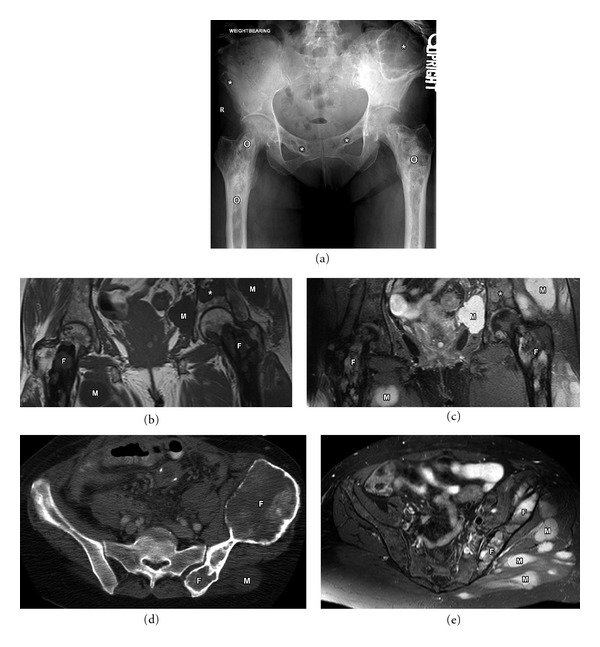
61-year-old female with Mazabraud syndrome (polyostotic fibrous dysplasia and soft tissue myxomas (M)). Plain film (a) demonstrates multiple lucent lesions with ground glass matrix in the pelvis (asterisk) and long predominantly sclerotic abnormality in bilateral femurs (circle). Fibrous dysplasia is often described as a long lesion in the long bone. Coronal T1 (b) and STIR (c) MRI images demonstrating multiple fibrous dysplasia (F) lesions. Note that some of these maintain low to intermediate signal intensity on both T1 and T2 images (asterisk). Axial CT (d) and axial T2 fat sat (e) MRI images demonstrating contour abnormality of the left ilium with ground glass matrix and focal bone expansion (F). Multiple T2 bright myxomas (M) are noted in the gluteal musculature.
